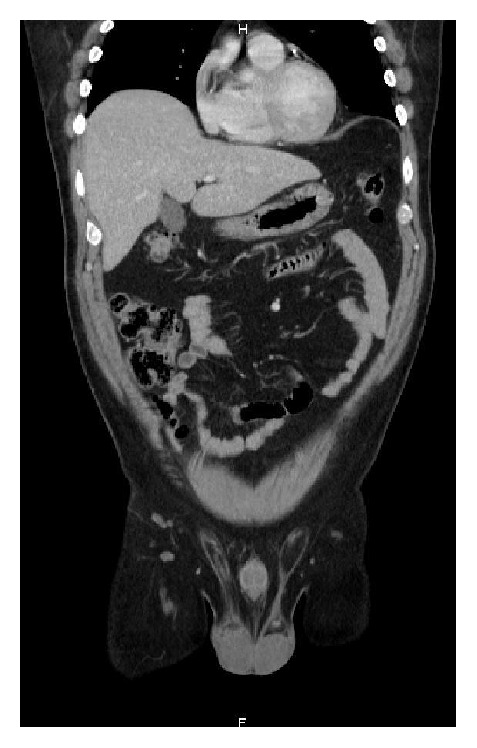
Comparative contrast CT scan after 1 month.
